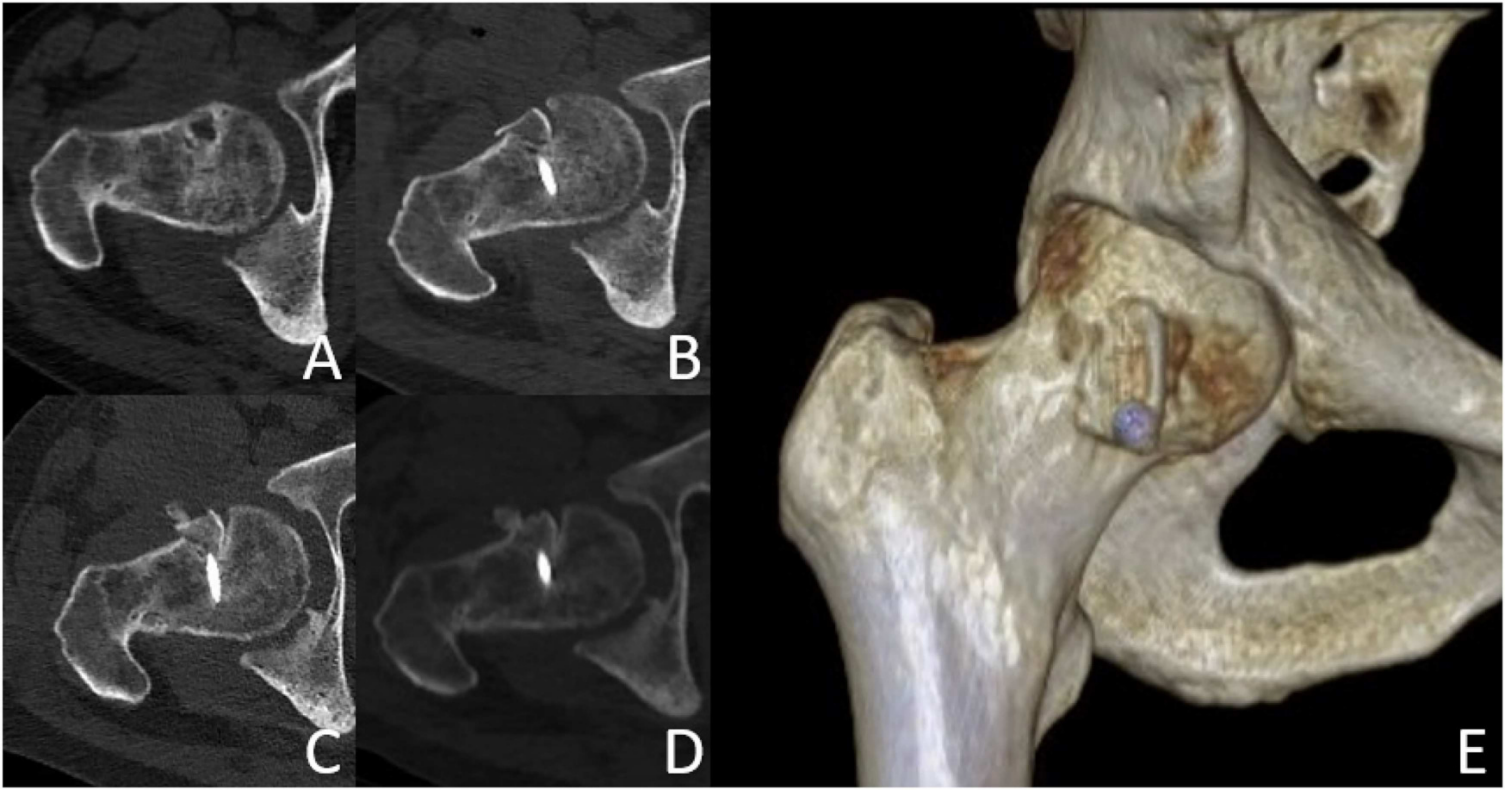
Follow-up CT and 3D reconstruction images. Preoperative CT cross-section (A); CT cross-section 1 day after surgery (B); CT cross-section 3 months after surgery (C); CT cross-section 6 months after surgery (D); 3D reconstruction image (E). CT images showed sufficient filling of the bone graft area, stable shape of the femoral head, and no further collapse.
